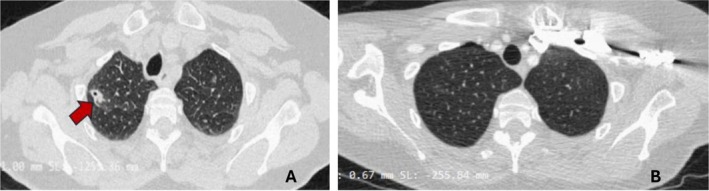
(A): Chest CT showed a partially cavitated nodule in the right upper lobe (red flag). (B): Complete regression of the upper right lobe nodule at 1 month follow‐up chest CT.
